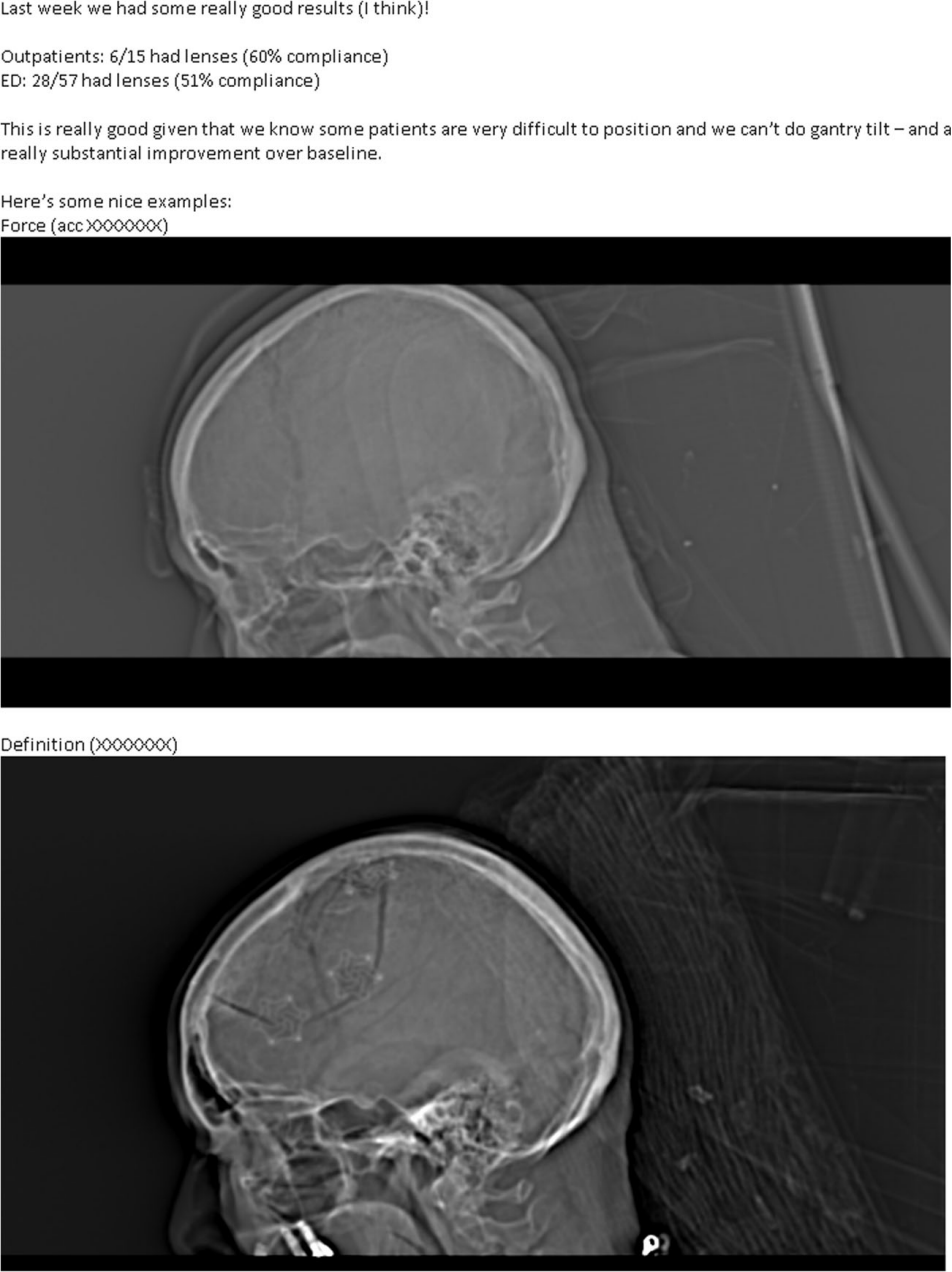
Representative weekly feedback e-mail with statistics, constructive criticism, and representative scout images showing optimal positioning for both computed tomography (CT) scanners
